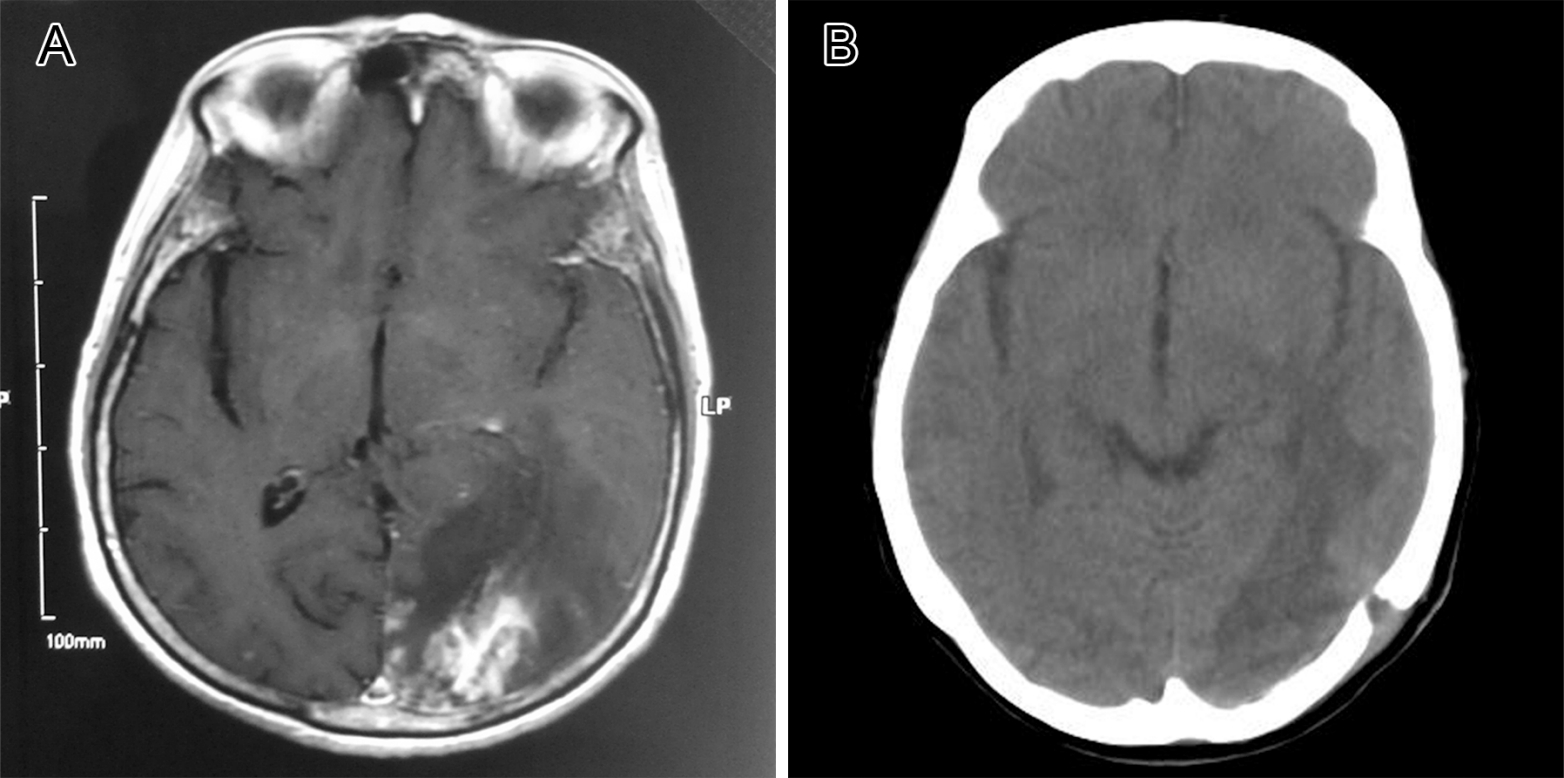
Comparison of brain imaging before and after treatment. (A) MRI before surgery showing an intracranial occupying lesion in the left occipital lobe, with edema and mottled nodular linear enhancement in the surrounding region. (B) CT result three months after surgery showing the disappearance of the nidus and fading of the edema. CT, computed tomography.
